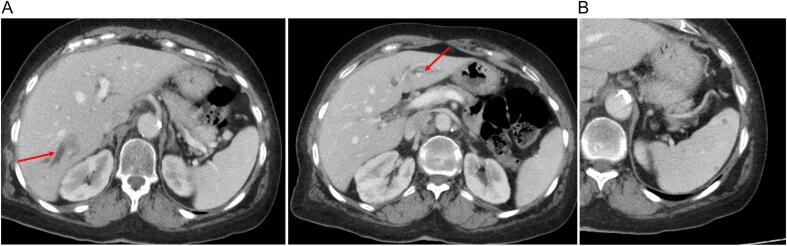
A: Axial abdominal CT scan with portal time injection showing a dilation of biliary tracts of liver segments II and VI. B: Axial abdominal CT scan with portal time injection showing multiples low dense lesion of the spleen.
